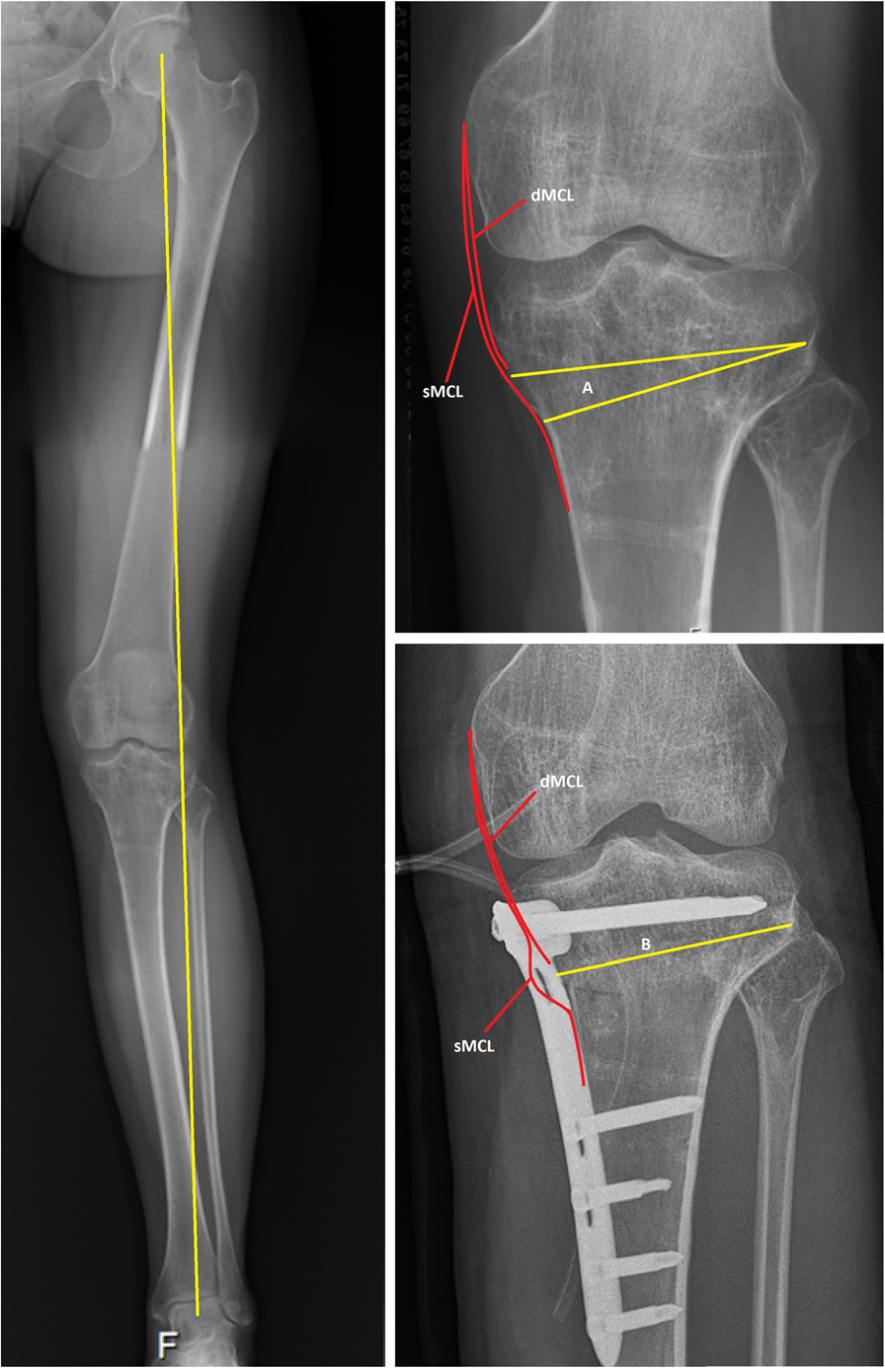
Preoperative and postoperative radiographs of the osteotomy site in relation to the medial collateral ligament. Subscript: The left figure shows a pre-operative long-leg radiographs with valgus malalignment. The right figures show a preoperative (above) and postoperative (below) radiograph of the knee. The osteotomy site (A and B) is below the insertion of the deep medial collateral ligament (dMCL). In the postoperative situation a pseudo laxity of the superficial medial collateral ligament (sMCL) is created
